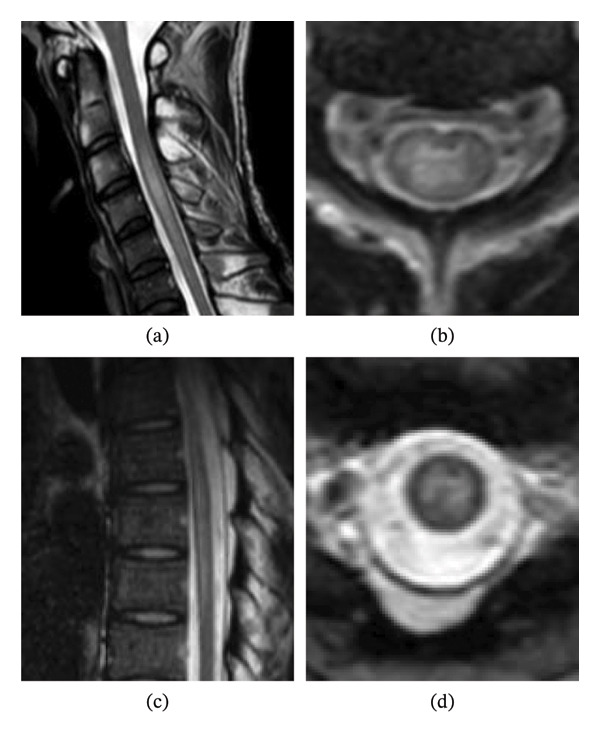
Magnetic resonance imaging (MRI) of spinal cord. T2‐weighted images showed intramedullary high‐intensity lesions with swelling, at C3–C5 (a, b) and Th6–Th8 (c, d) spinal levels ((a, c) sagittal views; (b, d) axial views).
